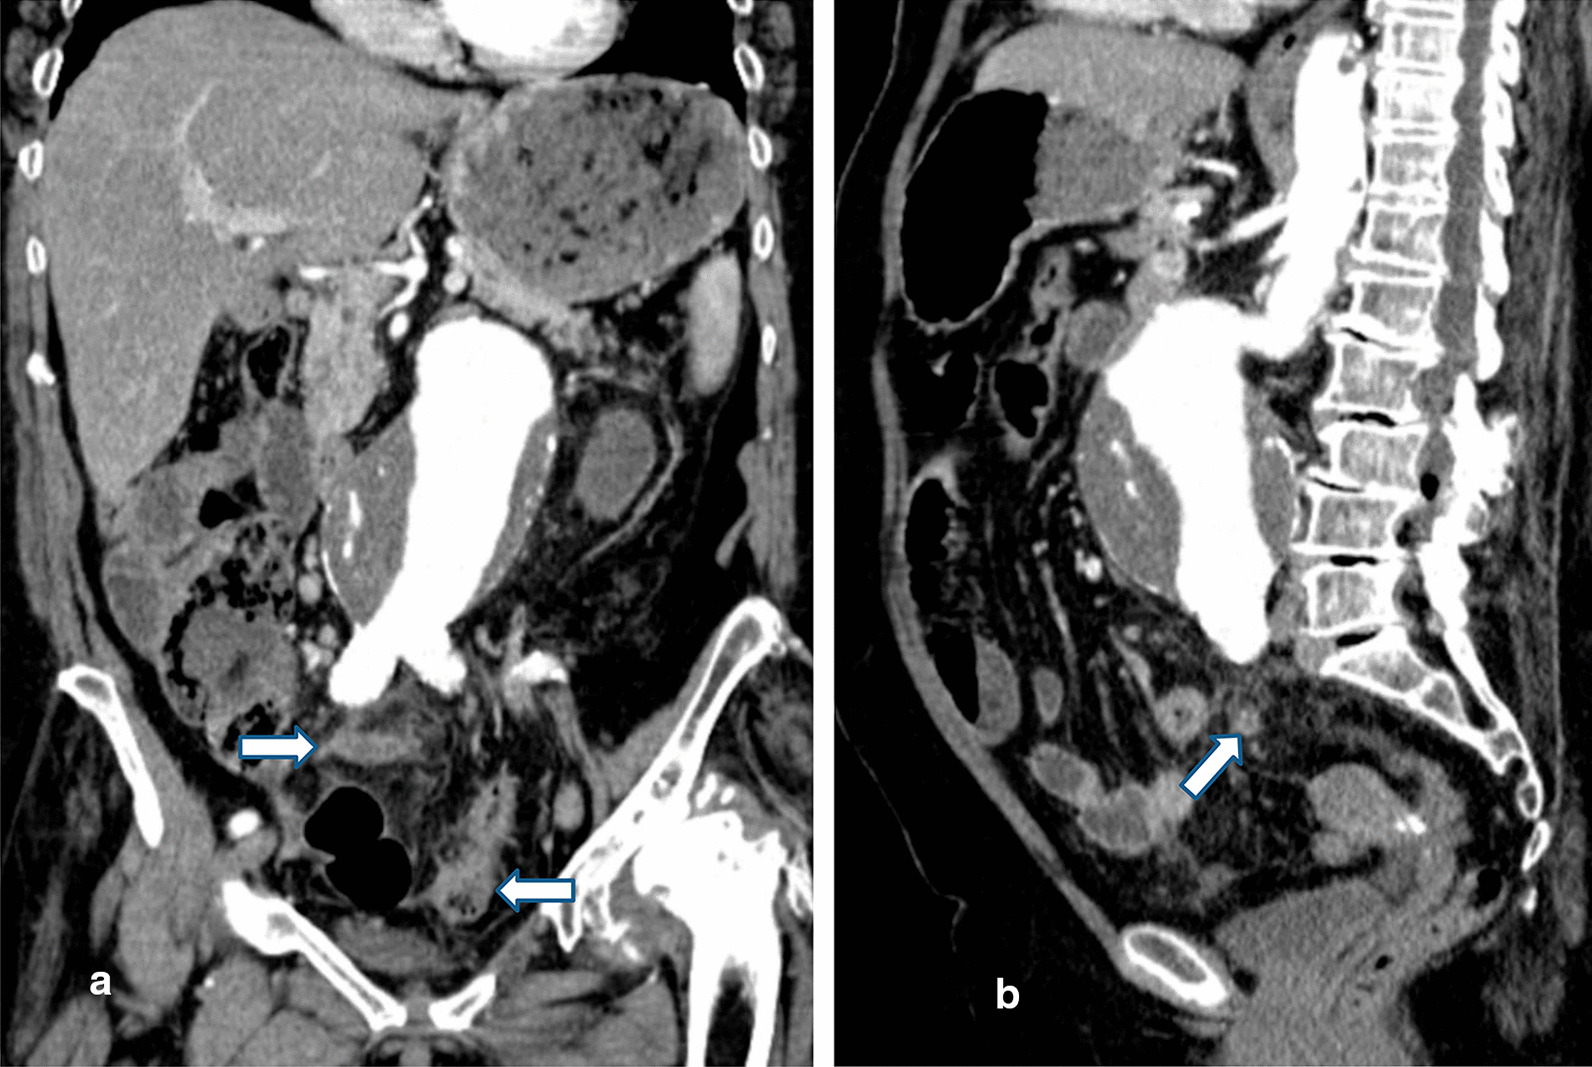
Preoperative computed tomography (coronal and sagittal sections): a inflamed appendix and increased echogenicity of adjacent fat (arrows). b Thickening of the sigmoid wall and slight increase in the echogenicity of the adjacent fat (arrow)
